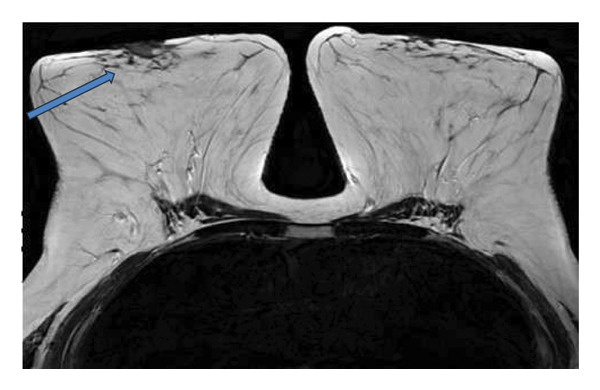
The patient (the same as in Figure 1) underwent breast ce‐MRI. The T2‐weighted sequence shows a right retro areolar nodular lesion containing a small fluid–debris collection (arrow in 2). The post‐contrast T1 sequence demonstrates a mass‐like enhancement in the right retro areolar region extending to the nipple, with a focal area of low signal intensity surrounded by enhancing margins, suggestive for a breast abscess (arrow in 3).
